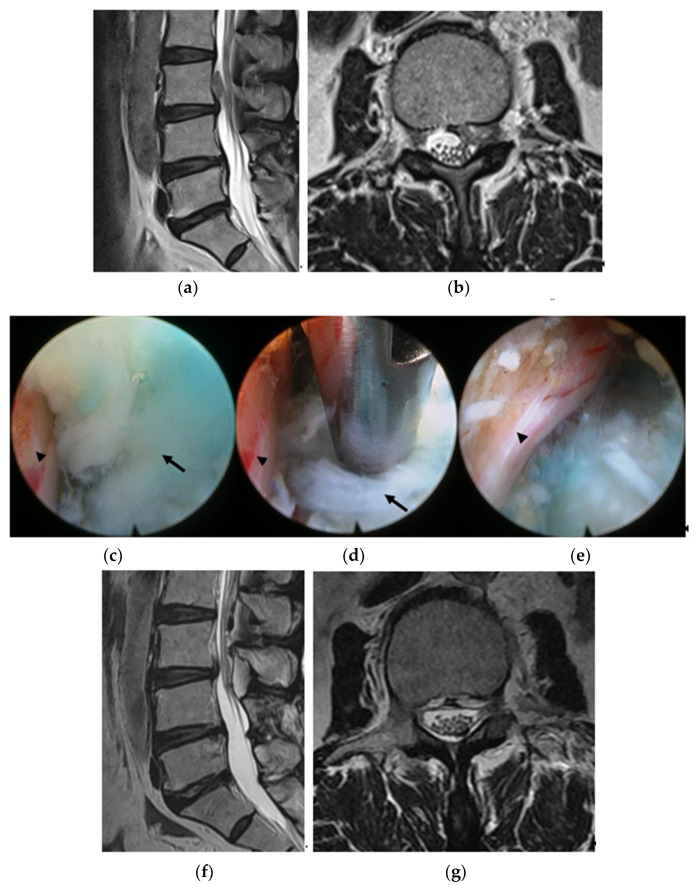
A 45-year-old man presenting with radiating pain in the left buttock and anterior thigh. Magnetic resonance imaging (MRI) revealed disc herniation with upward migration zone type C at L3–L4 (a,b). Ruptured disc particles were removed using the full endoscopic transforaminal approach (arrow: ruptured disc particles, arrow head: left side L3 nerve root, (c,d). After the full endoscopic transforaminal approach, the left side L3 nerve root was decompressed (e). A postoperative MRI showing that migrated ruptured disc particles were completely removed (f,g).
